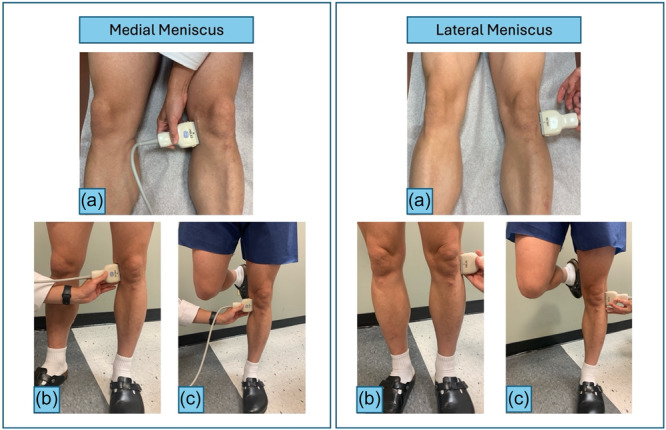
Positioning of the patient for ultrasound sonographic measurements. (a) Supine position, (b) Bipodal stance, (c) Unipodal stance. Meniscus extrusion was captured on ultrasound under these three loading conditions for both the medial and lateral meniscus.
